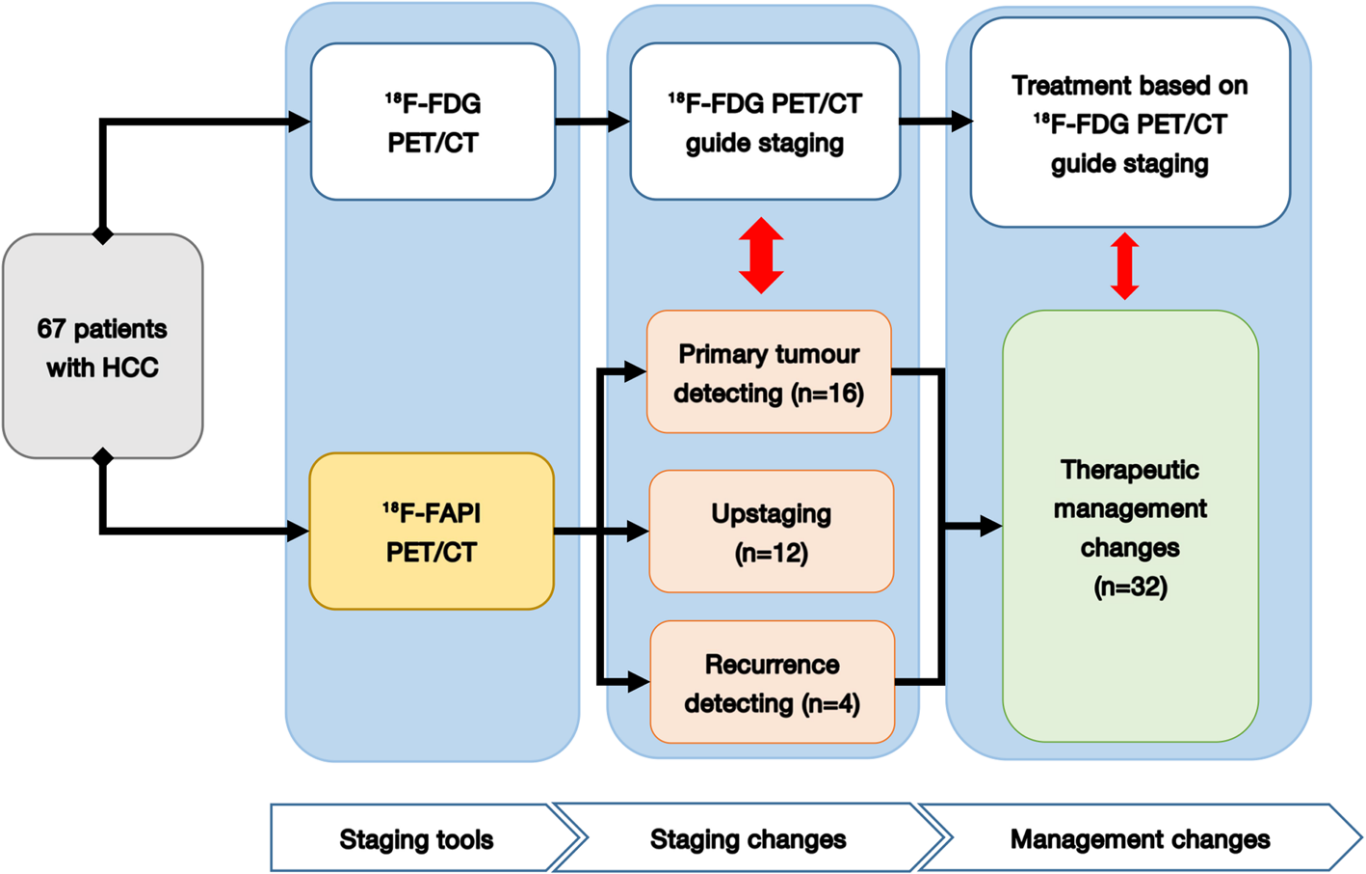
Overview of impact of ^18^F-FAPI PET/CT on staging and therapeutic management in HCC, therapeutic management was altered in 31 of 67 individuals
